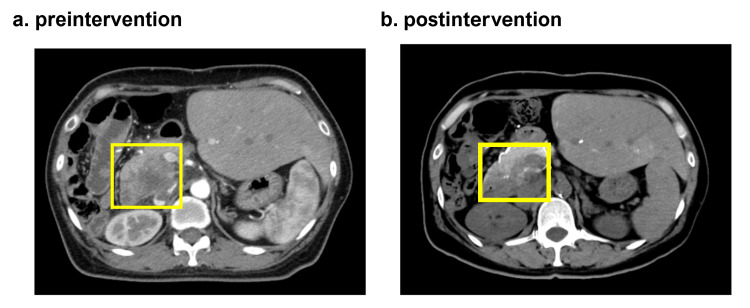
(a) Contrast computed tomography (CT) image of a woman with postoperative cholangiocarcinoma and pulmonary metastasis of pancreatic cancer taken before CDDP therapeutic intervention. A mass was observed in her pancreatic head after partial hepatic right lobe resection. (b) Her CT angiography image taken with contrast from the arteria hepatica propria one week after the preintervention image (a) was taken. Her pancreatic head tumor was observed to have shrunk. CDDP, cisplatin
